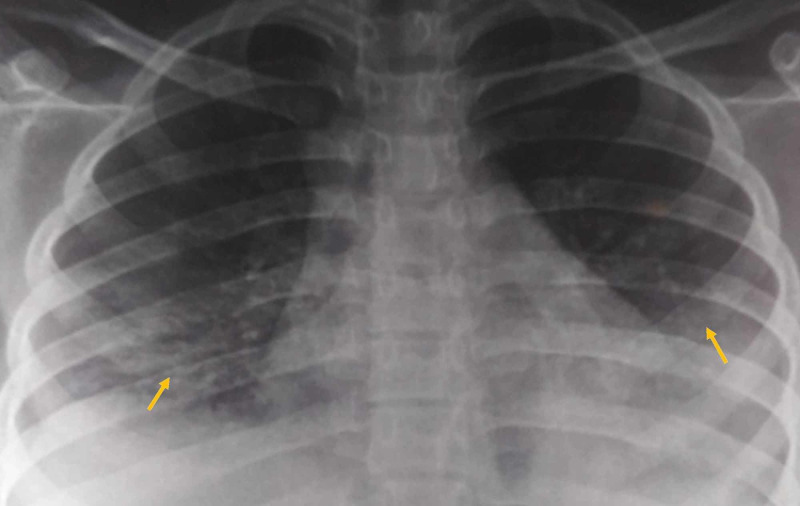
Chest x-ray posteroanterior (PA) view showing bilateral patchy infiltrates
